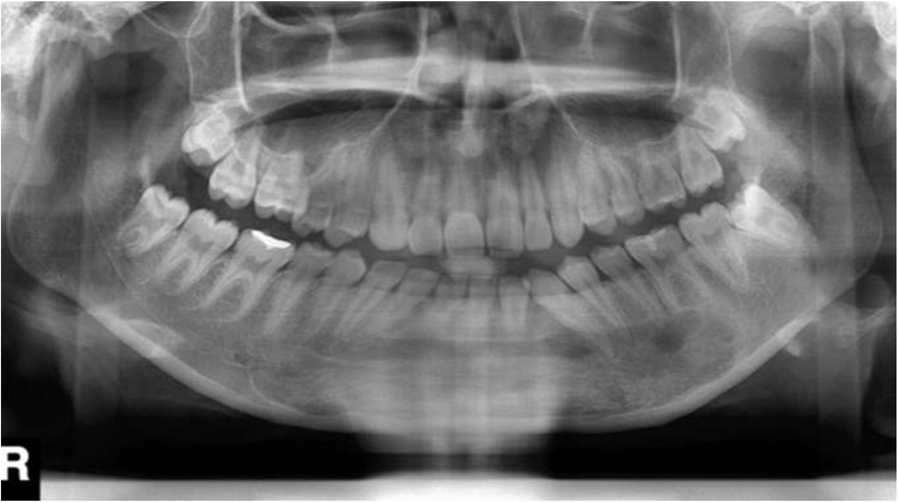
Panoramic radiographs after surgery. The dentition of the displaced teeth at the initial visit is restored somewhat to its original state
